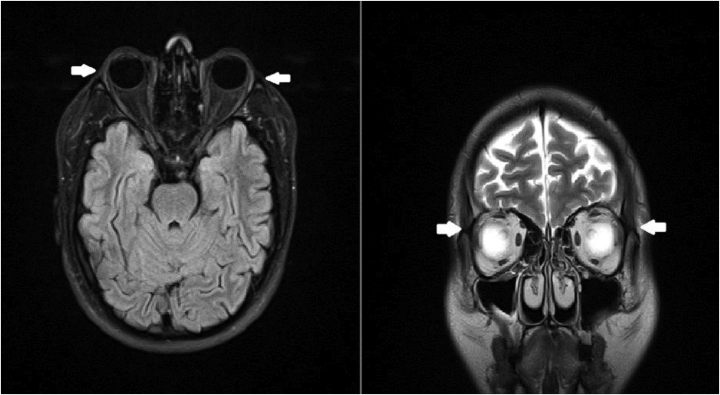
Brain MRI without contrast showing bilateral atrophic lacrimal glands with empty lacrimal fossa (white arrows).
